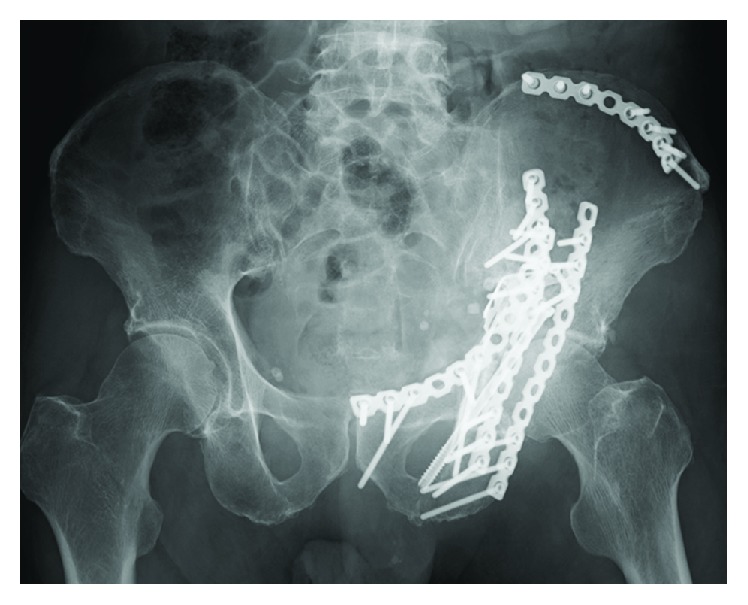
Postoperative radiographs. Both columns have been reduced and plated.
